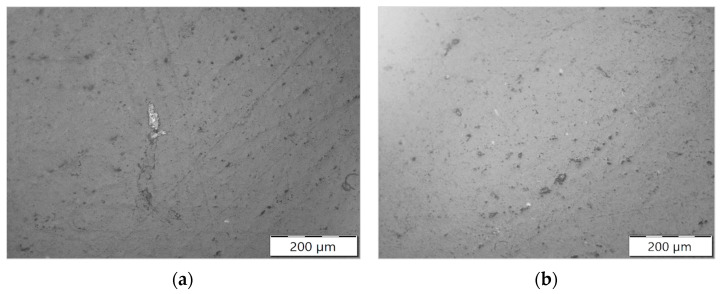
SEM images of the nano compounds. (a) Traditional extrusion compounding. (b) Ultrasound-assisted extrusion compounding.
